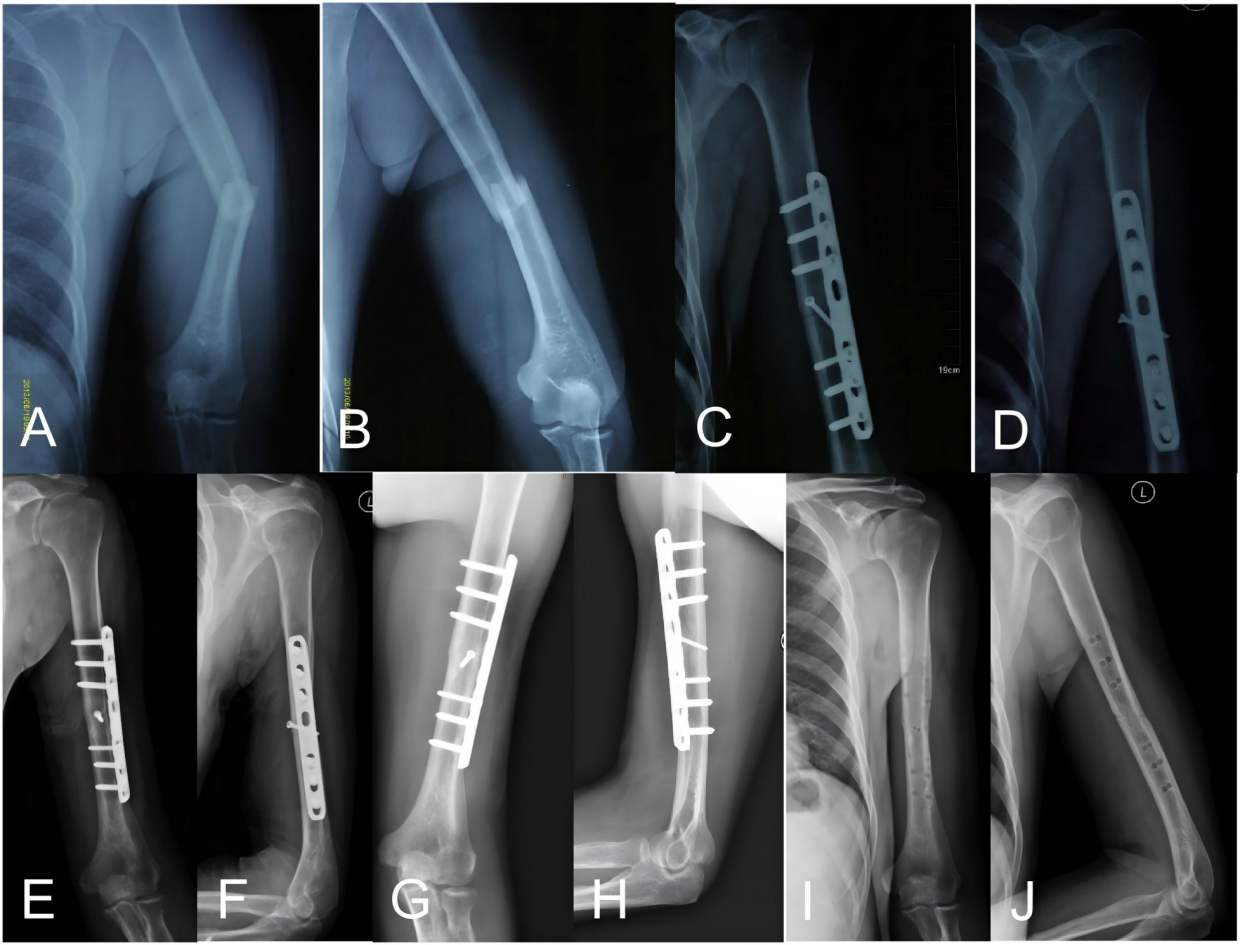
49-year-old male with a left humeral shaft fracture (AO/OTA A3) with angular shortening. (A,B) Preoperative radiographs. (C,D) Immediate postoperative radiographs after ORPO. (E,F) Radiographs at 12 weeks following surgery. (G,H) Radiographs at 24 months post-surgery. (I,J) Radiographs after removal of the internal fixation.
